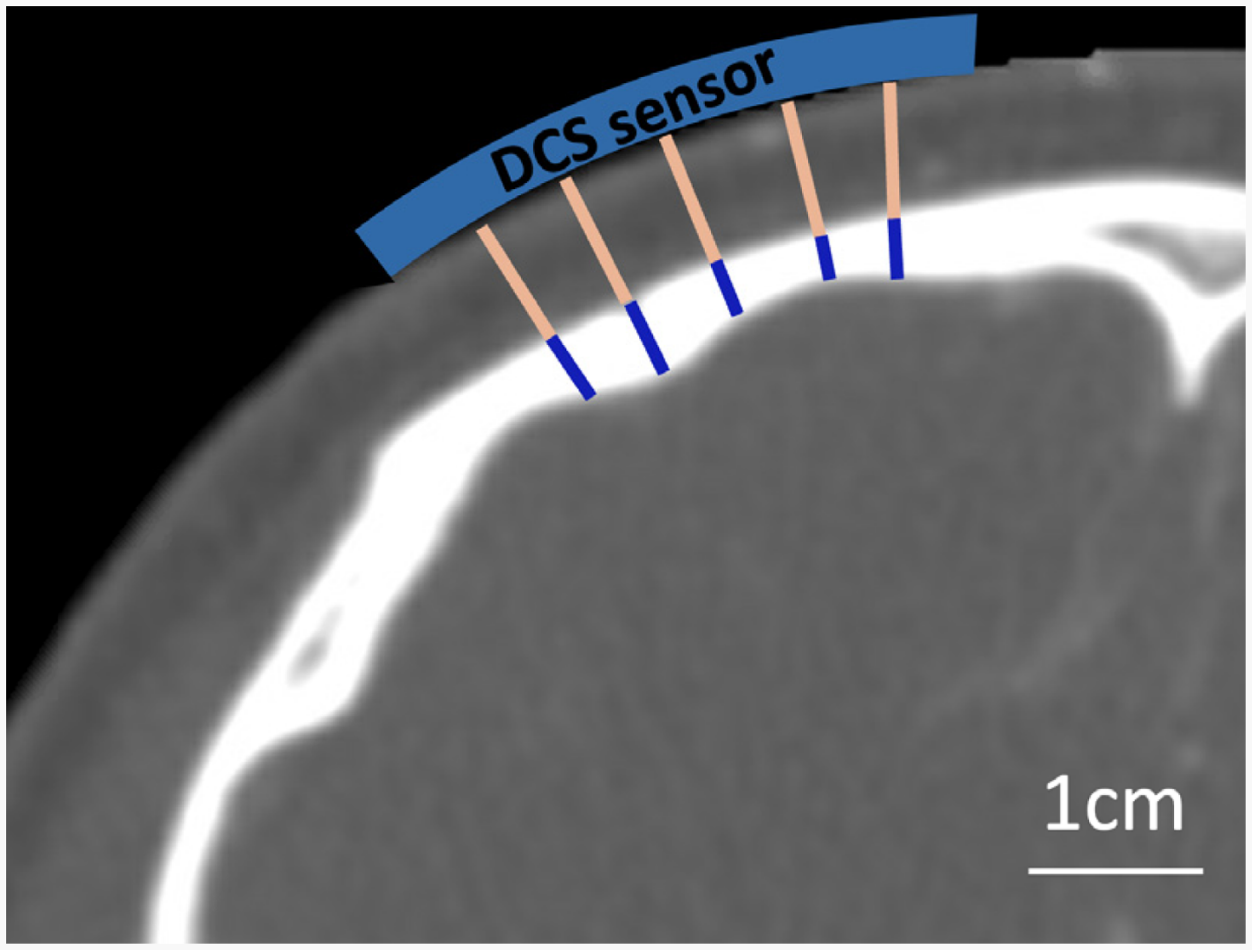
Representative measurements of scalp and skull layer thicknesses. Five separate measurements of scalp (in pink) and skull (in blue) layer thicknesses were made on an axial slice of the CT image. Measurements were made in the frontal region approximately where the DCS sensor was placed during monitoring.
